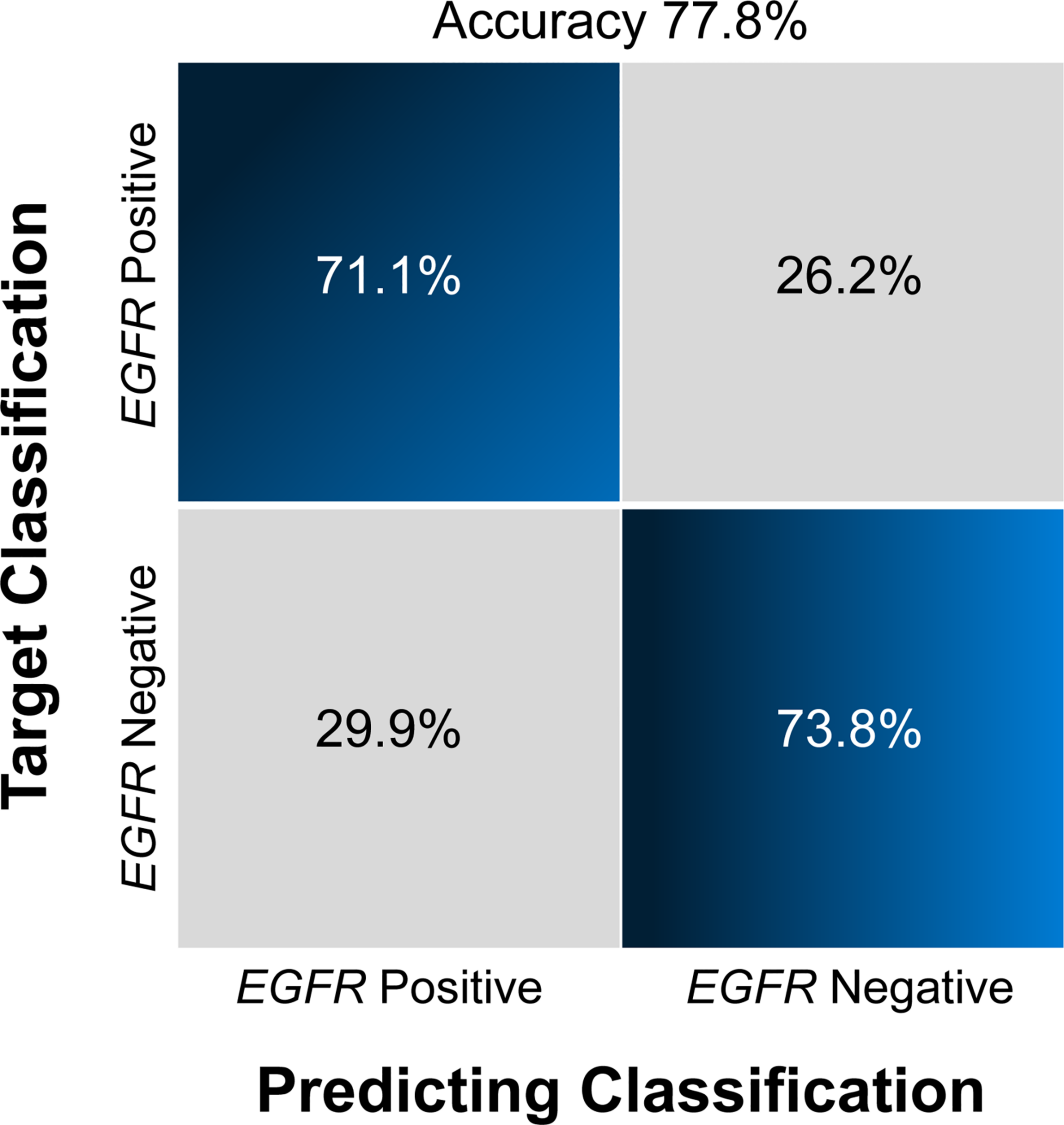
Confusion matrix (A) and ROCs (B) for the classification of *EGFR* mutation status in the test set The confusion matrix was generated using a combined model. The combined model appeared to achieve a higher AUC than any individual sequence, but the differences were not statistically significant. *EGFR*, epidermal growth factor receptor; ROC, receiver operating characteristics curve; AUC, area under the curve; T1CE, contrast-enhanced T1-weighted imaging; T2-FLAIR, T2 fluid-attenuated inversion recovery; T2WI, T2-weighted imaging; combination, combined model extracting features from the three sequences.
